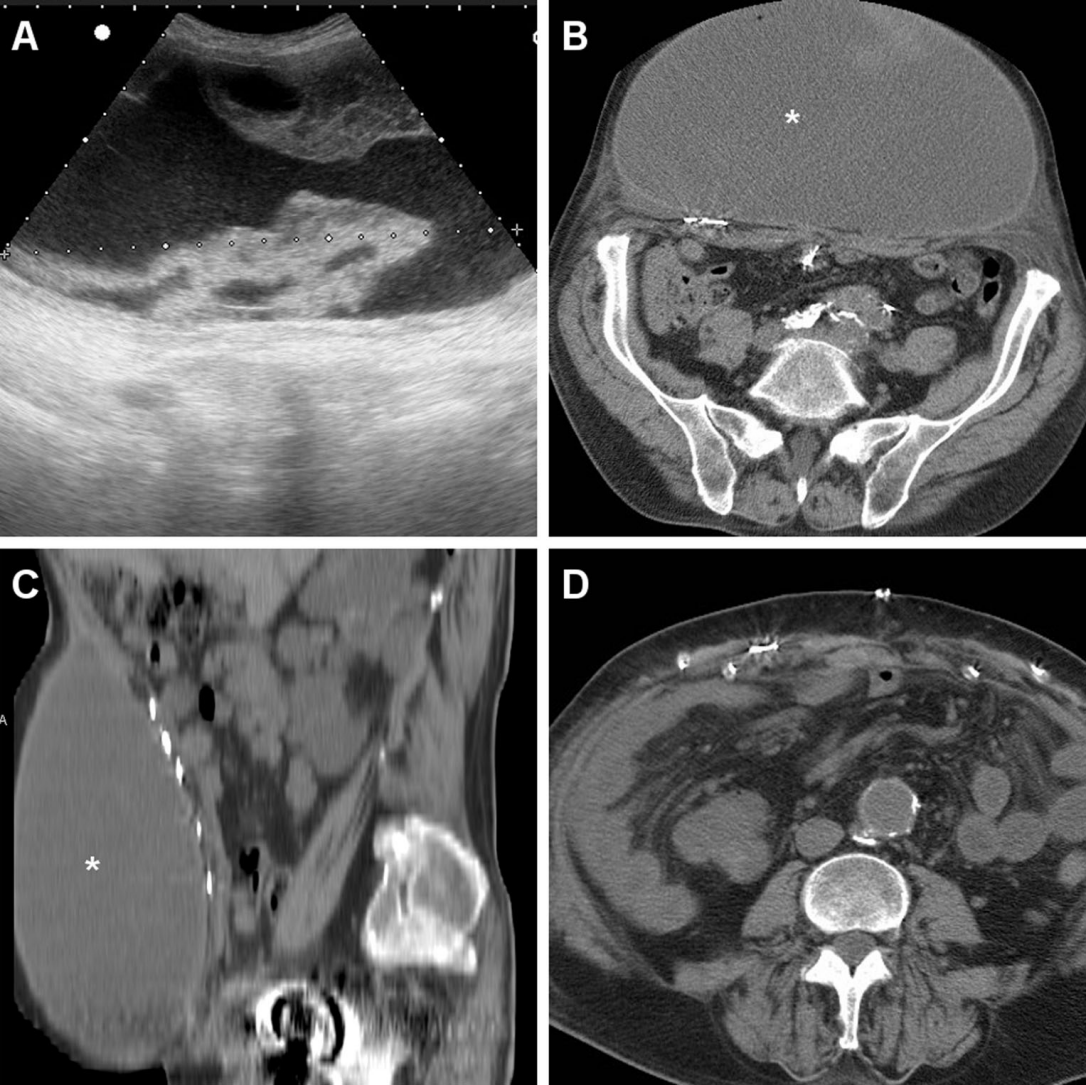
In a 75-year-old male, six weeks after VHR ultrasound (a) depicted a huge (25x11x18 cm) subacute haemorrhagic collection with mixed anechoic fluid, thick walls, and septa. Axial (b) and sagittal (c) unenhanced CT images depicted the liquefied haematoma (*) centered in the anterior abdominal wall, causing dislocation and compression of the PM indicated by the presence of metallic fixation clips. Repeated unenhanced CT (d) showed the postoperative status following drainage of two litres of serum and old blood
